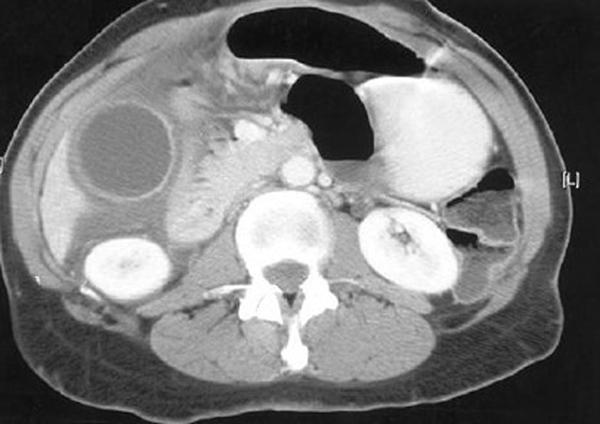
Abdominal computed tomography scan demonstrated a distended gallbladder with oedema and pericholecystic fluid.
